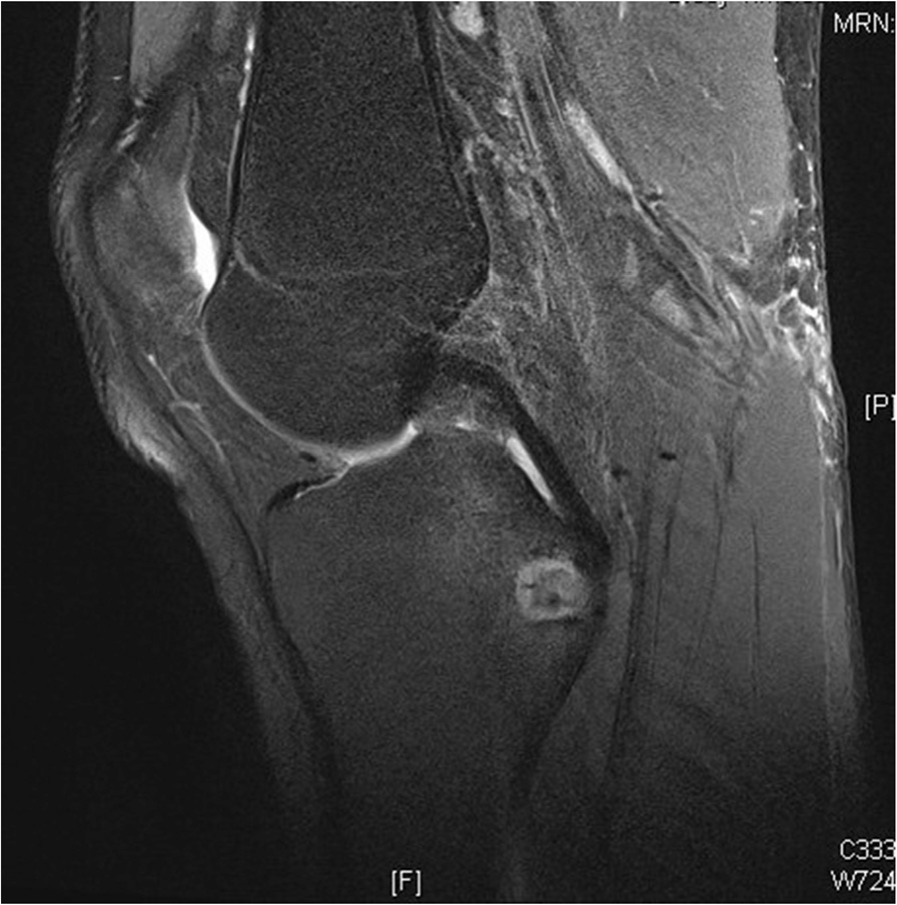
Magnetic resonance imaging scan of the left knee (sagittal view), follow-up examination (3 months postoperative)
